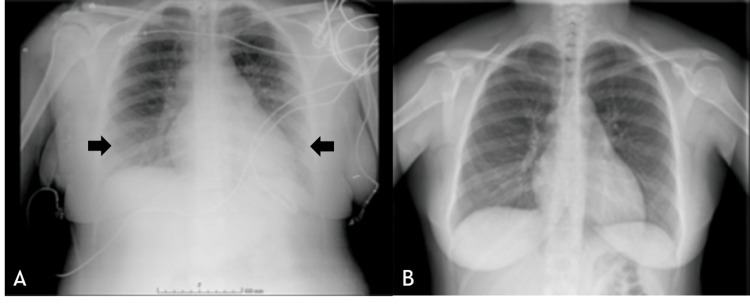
(A) Chest X-ray performed in PACU after delivery, showing marked hilar engorgement and diffuse bilateral infiltrate (black arrows); (B) chest X-ray performed five days after delivery, revealing no alterations. PACU, postanesthesia care unit
